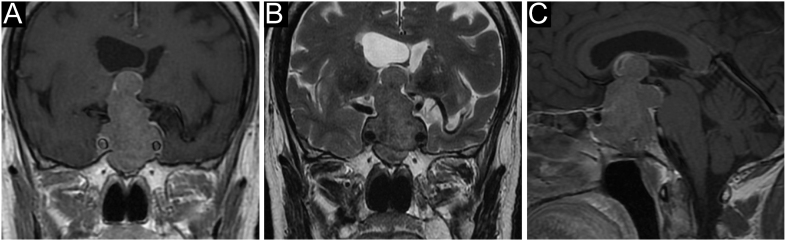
A giant invasive lactotroph PitNET in a 77-year-old man. This patient presented with fatigue, worsening headache and visual impairment, in the absence of significant medical history. Bitemporal hemianopsia was found at visual field examination, and brain MRI revealed a giant and invasive pituitary mass (maximal diameter 62 mm). Endocrine evaluation showed very high plasma PRL levels (20,000 ng/mL after dilution 1:100) with hypopituitarism. The patient was started on glucocorticoid and thyroxine replacement therapy with a low starting dose of cabergoline (CAB, 0.25 mg/week) and gradual titration up to 2 mg/week according to PRL values. A rapid clinical and visual response was observed, with a progressive reduction in tumour volume. PRL normalization was achieved within 1 year of treatment. The tumour went on shrinking to a small, intrasellar and cystic remnant (7 mm), and CAB was progressively reduced to a maintenance dose (1 mg/wk). The bio-clinical and radiological features remained stable over an 8-year follow-up. (A) Coronal contrast-enhanced T1-weighted scan, (B) coronal T2-weighted scan and (C) sagittal contrast-enhanced T1-weighted scan.
